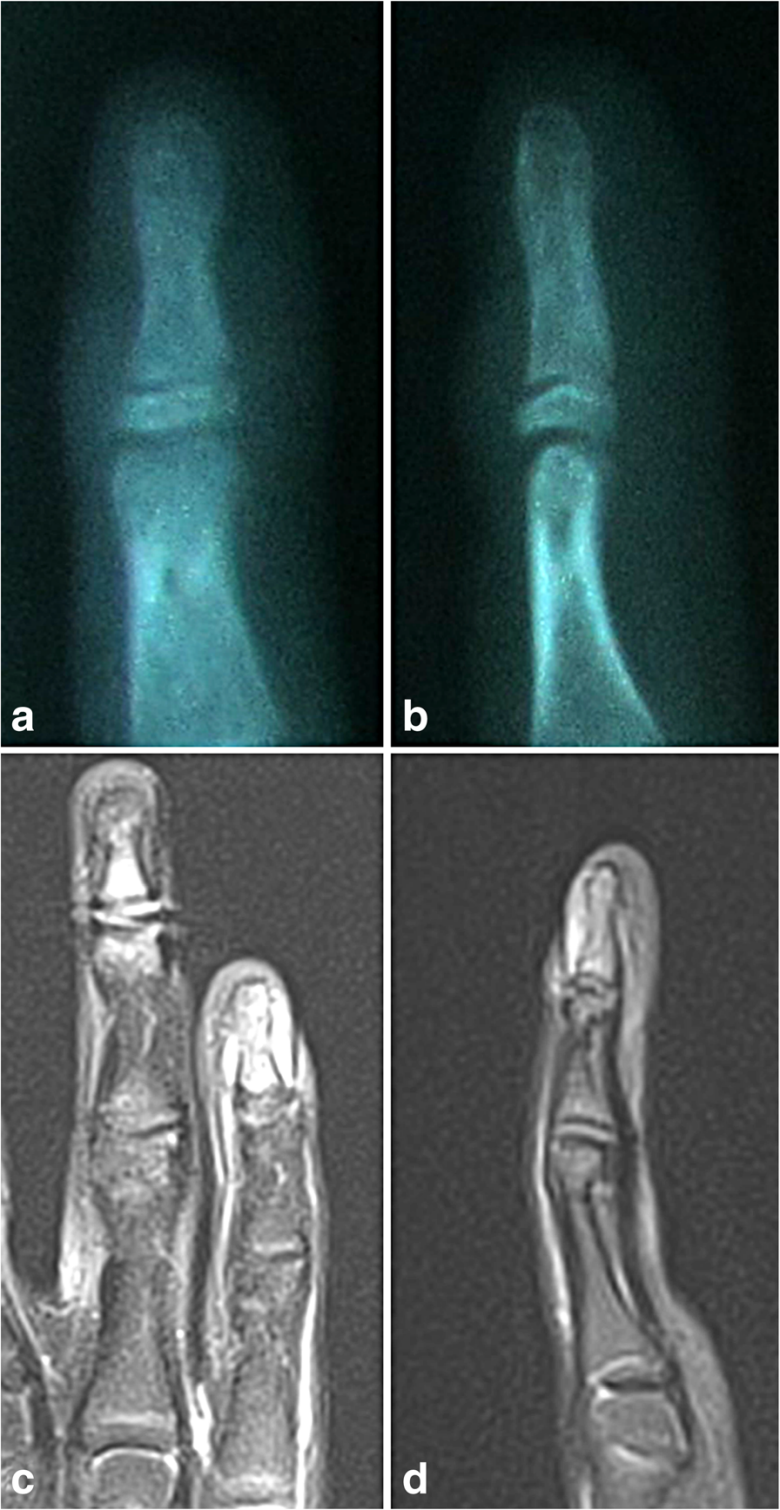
Initial radiographs of the left small finger distal phalanx in the anteroposterior (a) and lateral (b) view. Bone structure and mineralization is unremarkable and no signs of pathology are present. Magnetic resonance imaging of the left little finger in proton density fast spin echo sequence in the coronal (c) and sagittal (d) plane. Signal hyperintensity in the area of the distal phalanx, with no bone lesions is observed. These findings correlate with soft-tissue oedema medial, lateral and dorsal to distal phalanx
